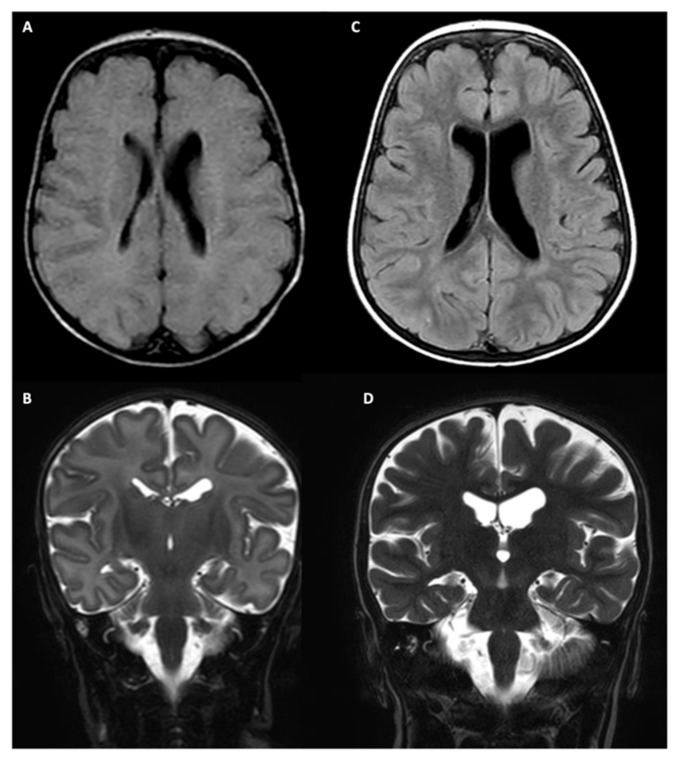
Brain MRI: (A,C) axial FLAIR; (B,D) coronal T2 sequencies. The patient’s brain MRI, performed at the age of 1 month, showing mild asymmetry of lateral ventricles; brain MRI of the same girl repeated at the age of 33 months, showing atrophic progression mainly affecting the white matter and the hippocampi.
